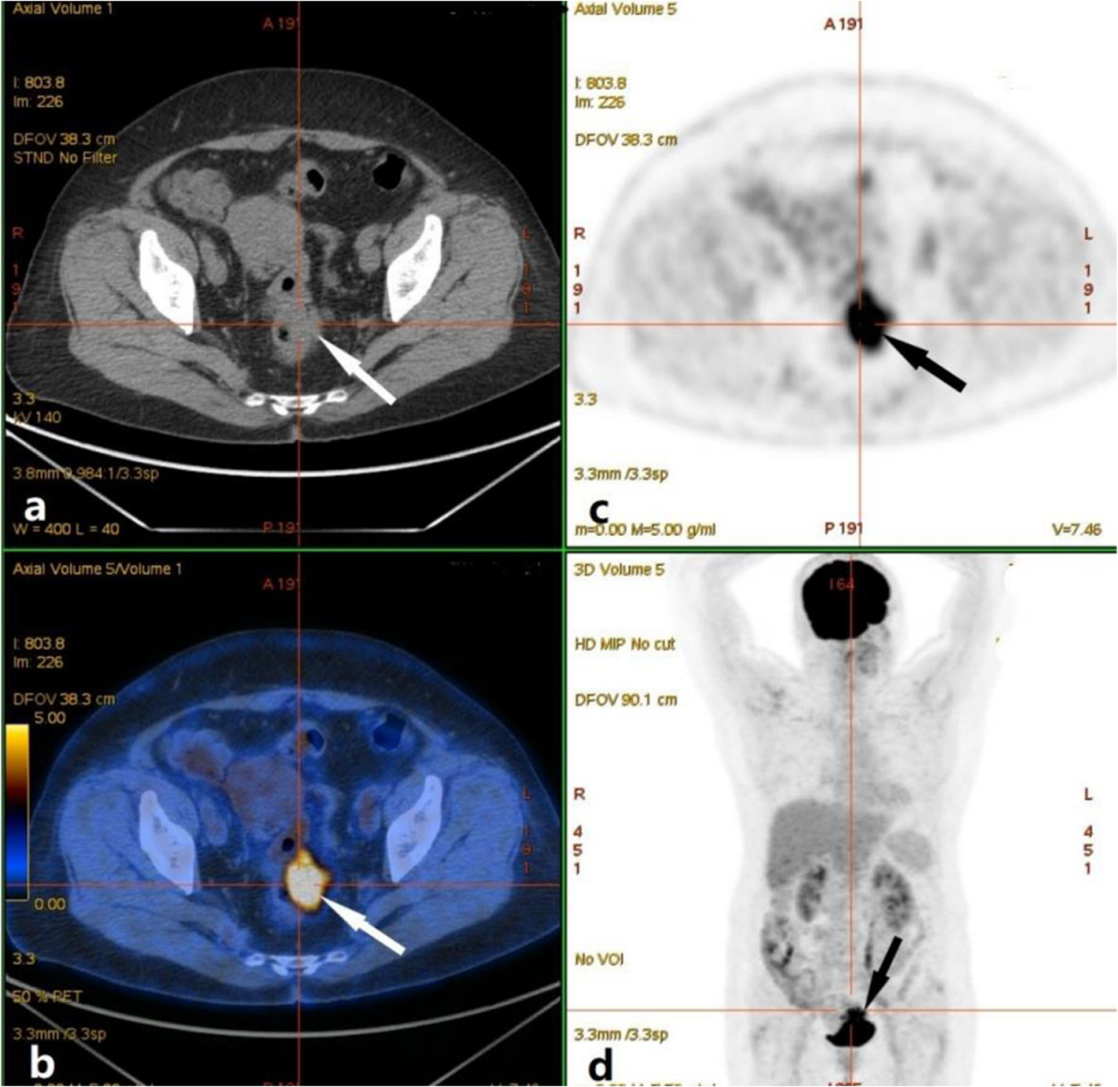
Positron emission tomography/computed tomography (PET/CT), showing a mass with fluorodeoxyglucose (FDG) uptake in the rectovaginal fossa. a CT image showing a cystic-solitary mass. b PET/CT image showing a mass with FDG uptake (maximum standardized uptake: 11.32). c, d FDG images
